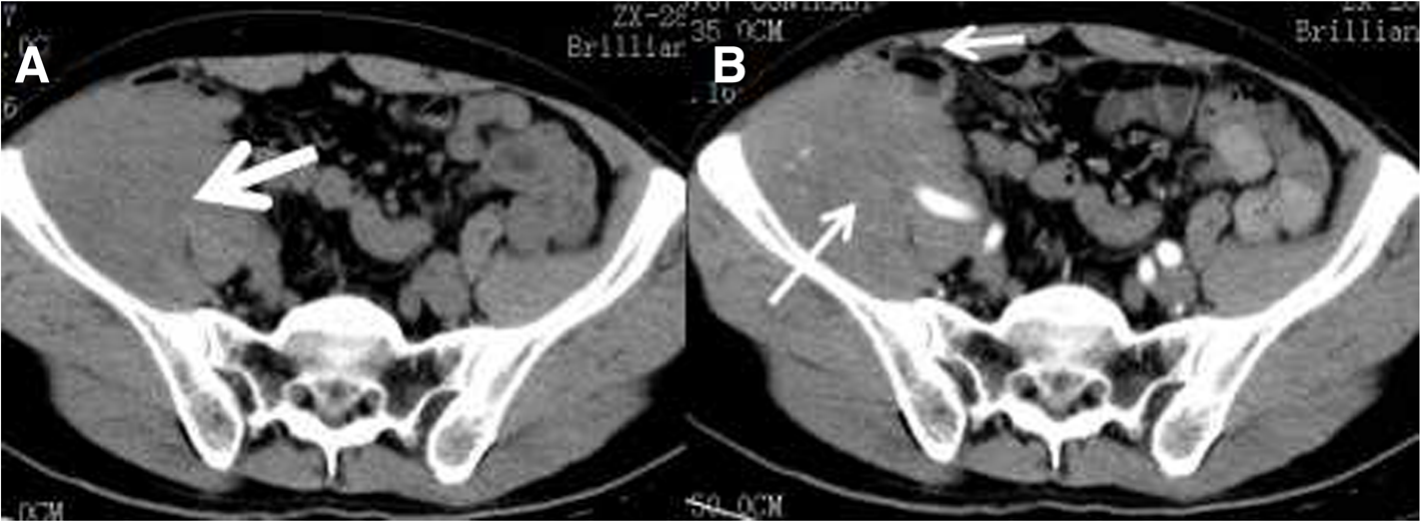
A right intra-abdominal undescended testis secondary seminoma. a A CT plain scan showed irregular soft tissue in the right iliac fossa (arrow). This tissue had a homogeneous density and a CT value of 38 HU. b In the enhanced arterial phase, the CT value of the lesion was 55 HU, and the blood vessels were embedded in the lesion. The boundary of the lesion and adjacent loop of bowel (short arrow)/iliacus (long arrow) were not clear, which was confirmed by biopsy at surgery
